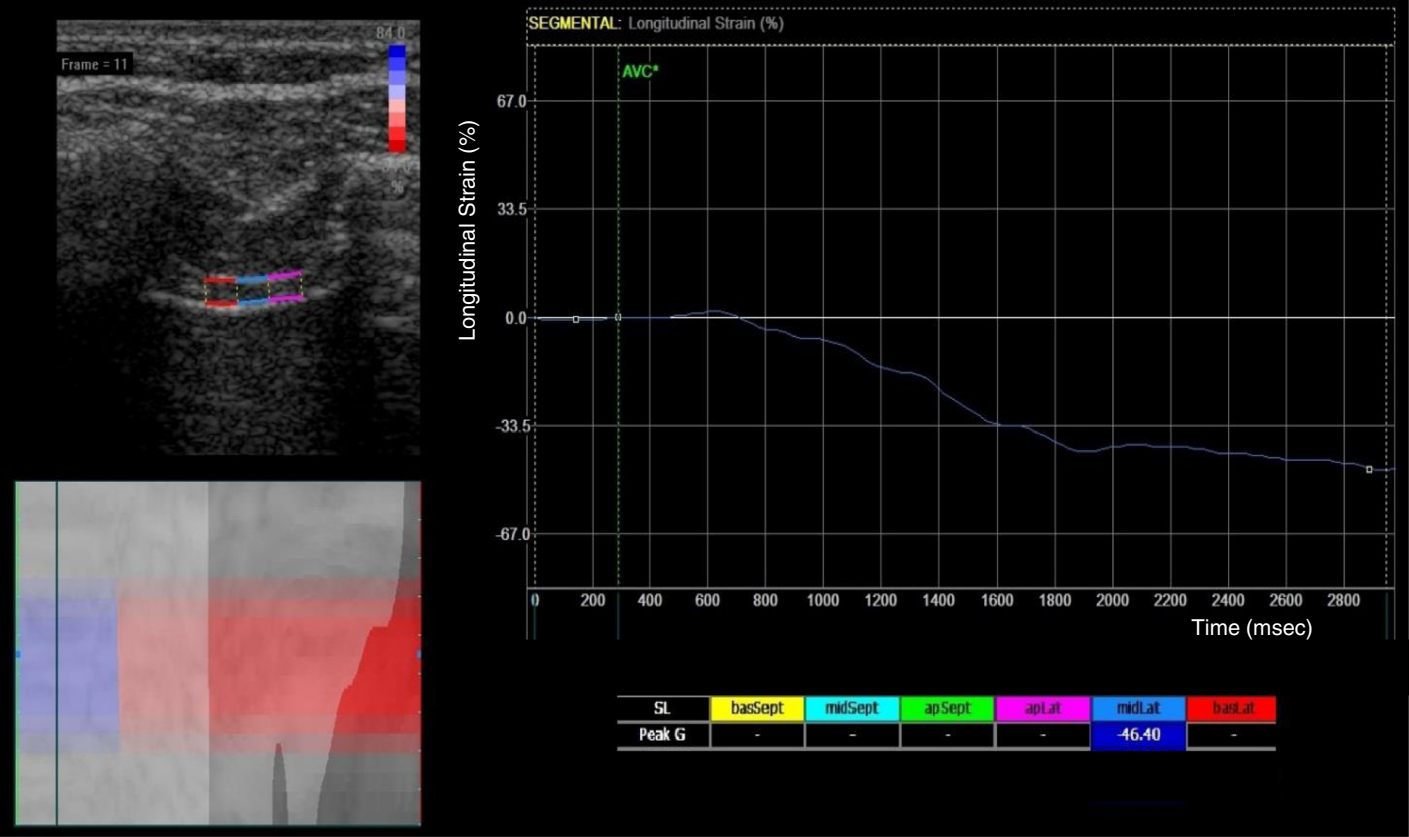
Longitudinal strain of diaphragm during inspiration. The x-axis represents time (millisecond), and the y-axis represents longitudinal strain (%). Strain is a measure of relative deformation and is a negative value. In this example, the central portion of the region of interest (depicted in blue in the ultrasound image at the upper-left corner) was traced and measured as – 46.4 % (displayed in the column below the x-axis). The more negative value means the higher degree of deformation (contraction)
